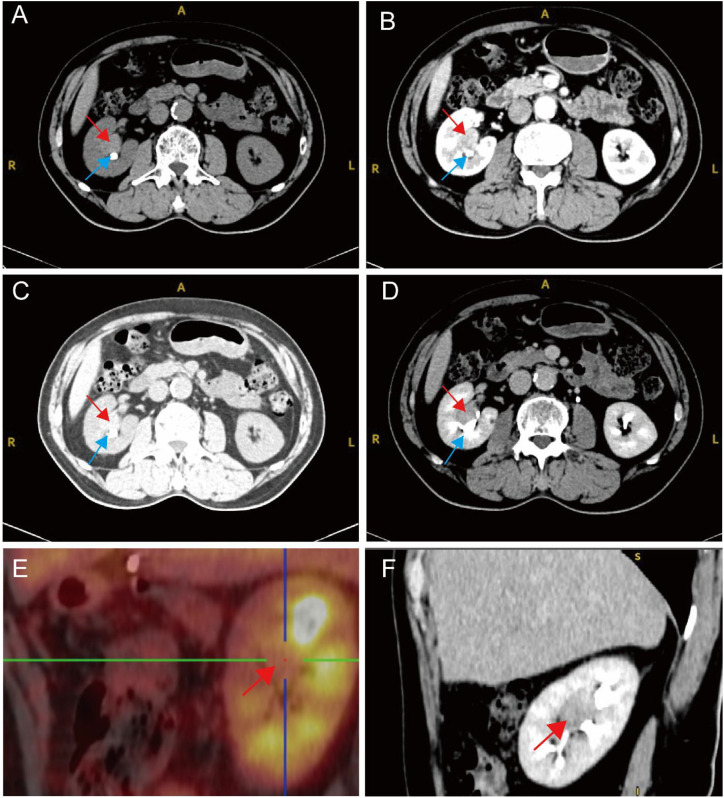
Imaging findings of the right renal mass. (A) Non-contrast CT (axial view) showing a nodular soft tissue density lesion in the right renal pelvis region measuring 25 mm × 17 mm, with well-defined borders and compression of surrounding adipose tissue (CT value: 51 HU). Red arrow indicates the mass; blue arrowhead indicates the renal pelvis calculus. (B) Contrast-enhanced CT in the arterial phase (axial view) demonstrating mild enhancement of the mass (57 HU). Red arrow indicates the mass; blue arrowhead indicates the renal pelvis calculus. (C) Contrast-enhanced CT in the corticomedullary phase (axial view) showing marked enhancement of the mass (102 HU). Red arrow indicates the mass; blue arrowhead indicates the renal pelvis calculus. (D) Contrast-enhanced CT in the excretory phase (axial view) demonstrating moderate enhancement of the mass (74 HU) with a filling defect in the right renal pelvis. Red arrow indicates the mass; blue arrowhead indicates the renal pelvis calculus. (E) PET-CT (sagittal view) revealing increased FDG uptake in the right renal hilar mass with SUVmax of 2.6, indicating increased glucose metabolism. Red arrow indicates the mass. (F) Contrast-enhanced CT in the excretory phase (sagittal view) showing opacification of the renal calyces with a filling defect in the renal pelvis region, demonstrating non-visualization of contrast material in the area occupied by the mass. Red arrow indicates the mass.
